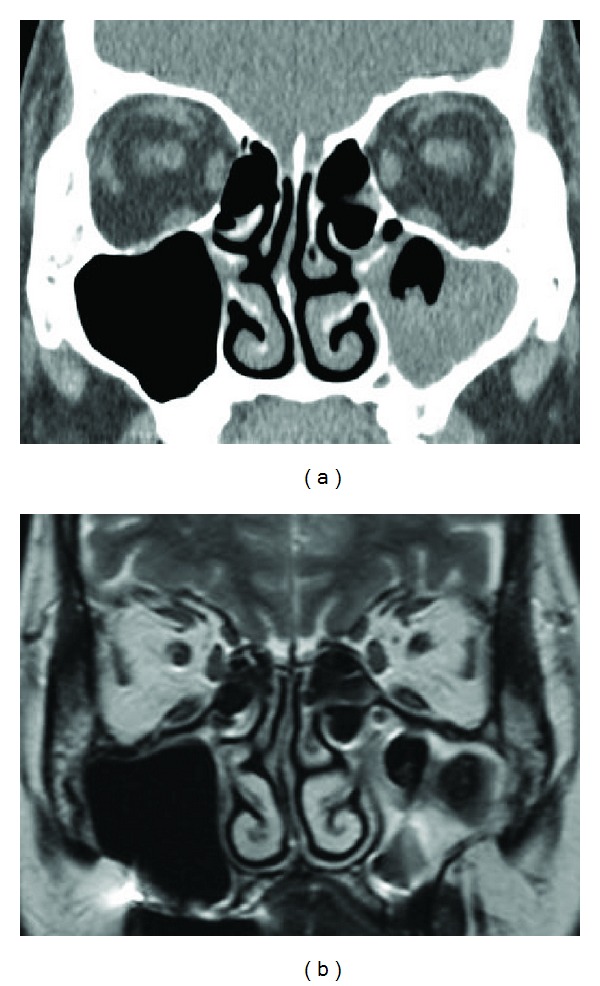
Representative case of fungus ball identifiable only on MR imaging. (a) Coronal CT scan with soft tissue density. Left maxillary sinus is filled with material. Irregular surface of the material suggests the possibility of fungus ball. High density spot is not seen. (b) Coronal T2-weighted MR image. Extremely low signal intensity to signal void indicates the presence of fungus ball.
